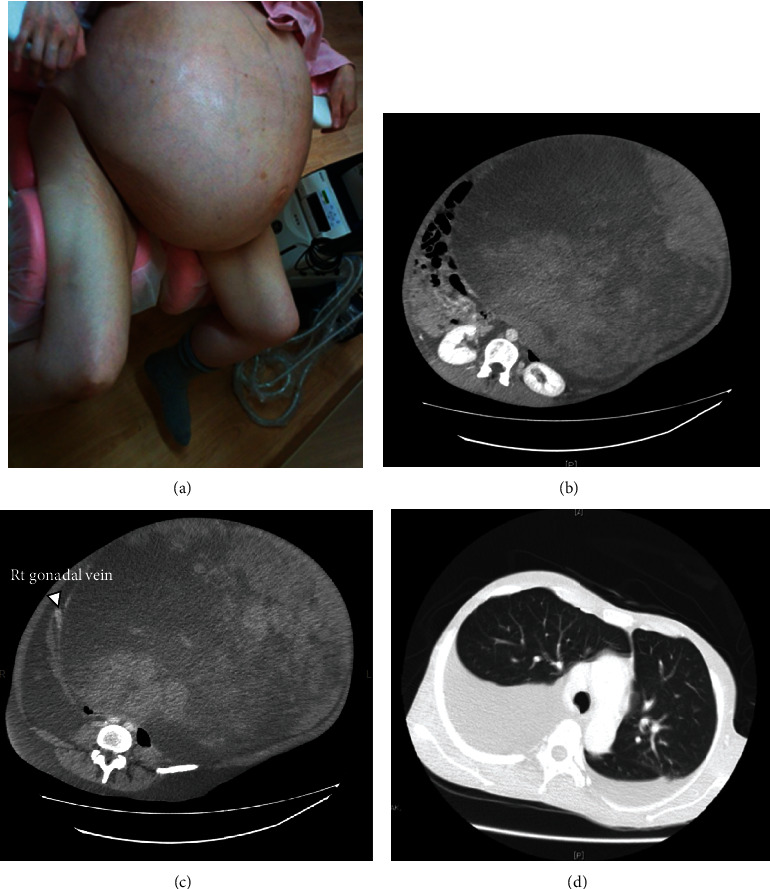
Physical and imaging test findings before operation. (a) Her abdomen was markedly distended, making it difficult for her stand by herself. (b, c, d) Findings of enhanced-computed tomography. (b) Majority of the mass was homogenous with a density of subcutaneous fat and no obvious hypervascular lesions. (c) The right gonadal artery and vein flow into the tumor. (d) The pleural effusion was detected in right thoracic predominance.
